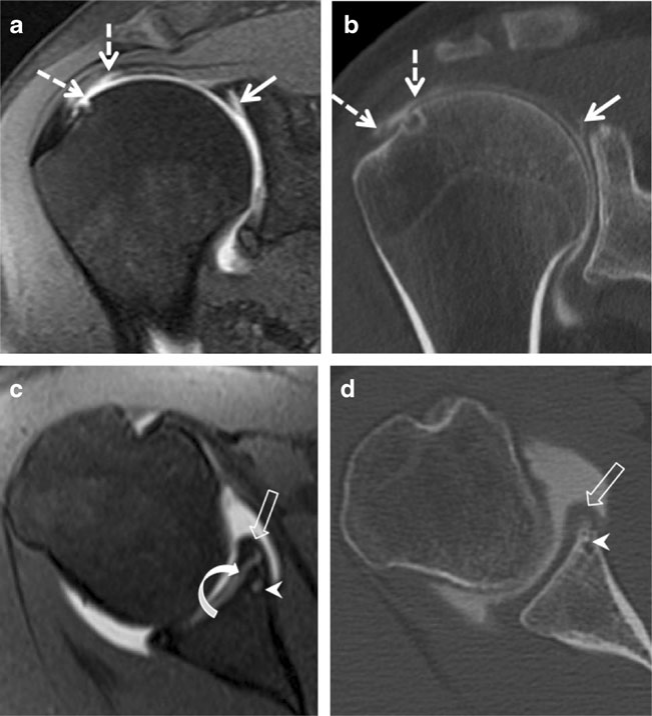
SLAP tear type V with rotator cuff lesion in a 38-year-old male athlete with right shoulder pain. a Coronal and c axial fat-suppressed T1-weighted MR arthrography and correspondent b coronal and d axial CT arthrography images show an intermediate undersurface tear of the anterior portion of the supraspinatus (*dashed arrows*). Note also the presence of a labro-bicipital tear extending across the biceps attachment (*curved arrow*) and inferiorly (*11*–*6 o’clock*). Hypertrophy (*empty arrow*) and linear tear (*curved arrow*) of the inferior labrum communicating with a small paralabral cyst (*arrowhead*) are noted. This constellation of findings is in keeping with SLAP V lesion
